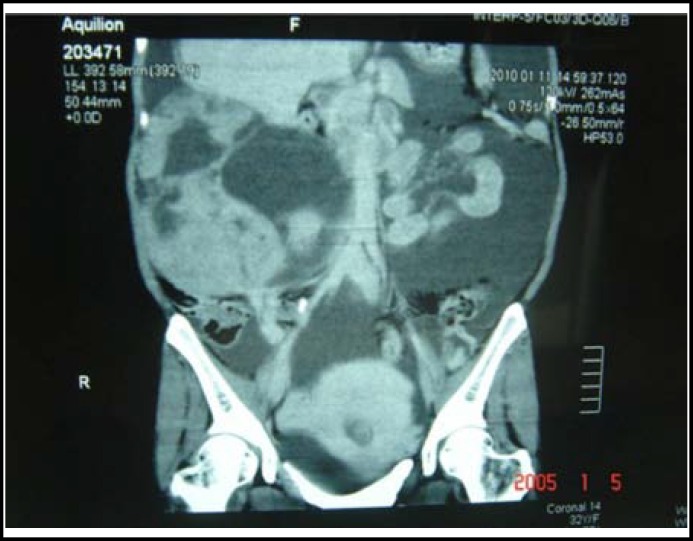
Computed tomography examination showed a right ovarian tumor with a size of 17.3 cm × 16.2 cm ×12.3 cm accompanied by significant abdominal and pelvic fluid
